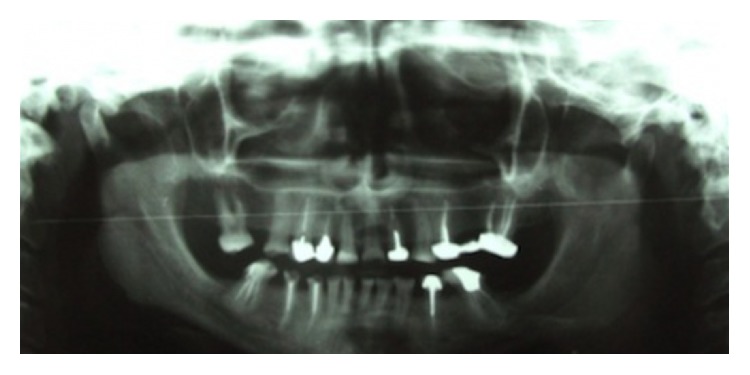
Panoramic radiographic image before treatment.
